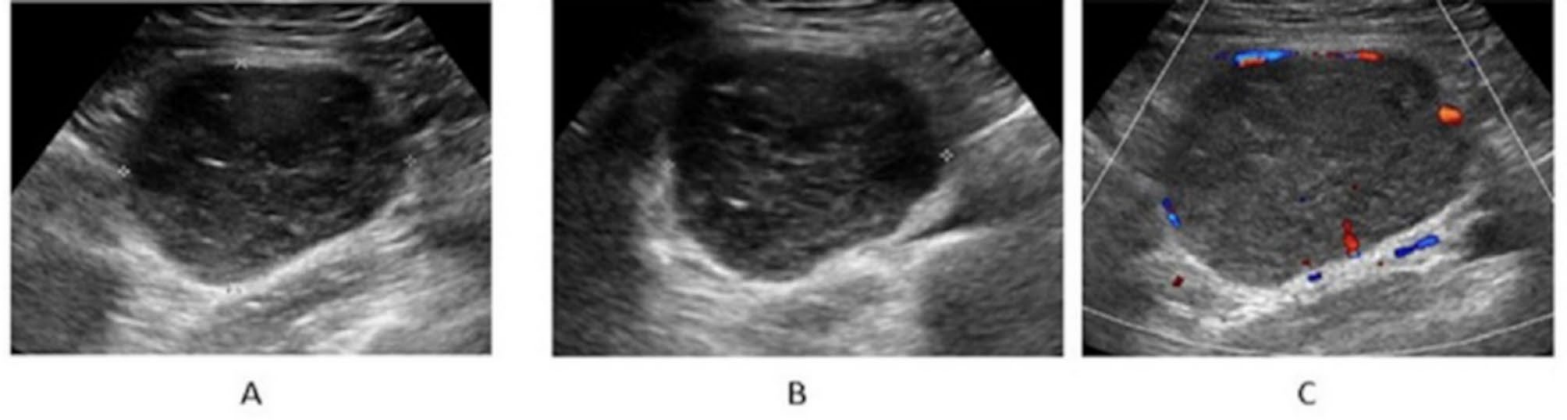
The US findings of an enlarged LN in a 50-year-old woman with a palpable mass in the right lower quadrant. The pathological diagnosis of the enlarged LN was the mixed type (A- B) The enlarged lymph node grayscale US. The retroperitoneal lymph node presented 7.8 × 7.2 × 6.6 cm in size. The LN showed complete effacement of the fatty hilum and a short linear hyper-echo within the LN. (C) The Color Doppler image. The LN showed moderate non-hilar color flow US, ultrasound; LN, lymph node; cm, centimeter
